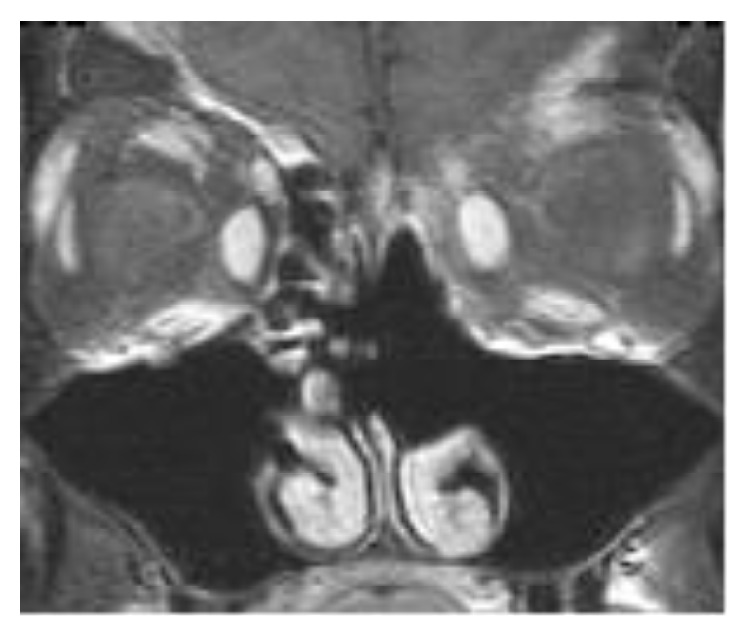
Coronal MRI 2 years after operation showing no residual or recurrent tumor.
